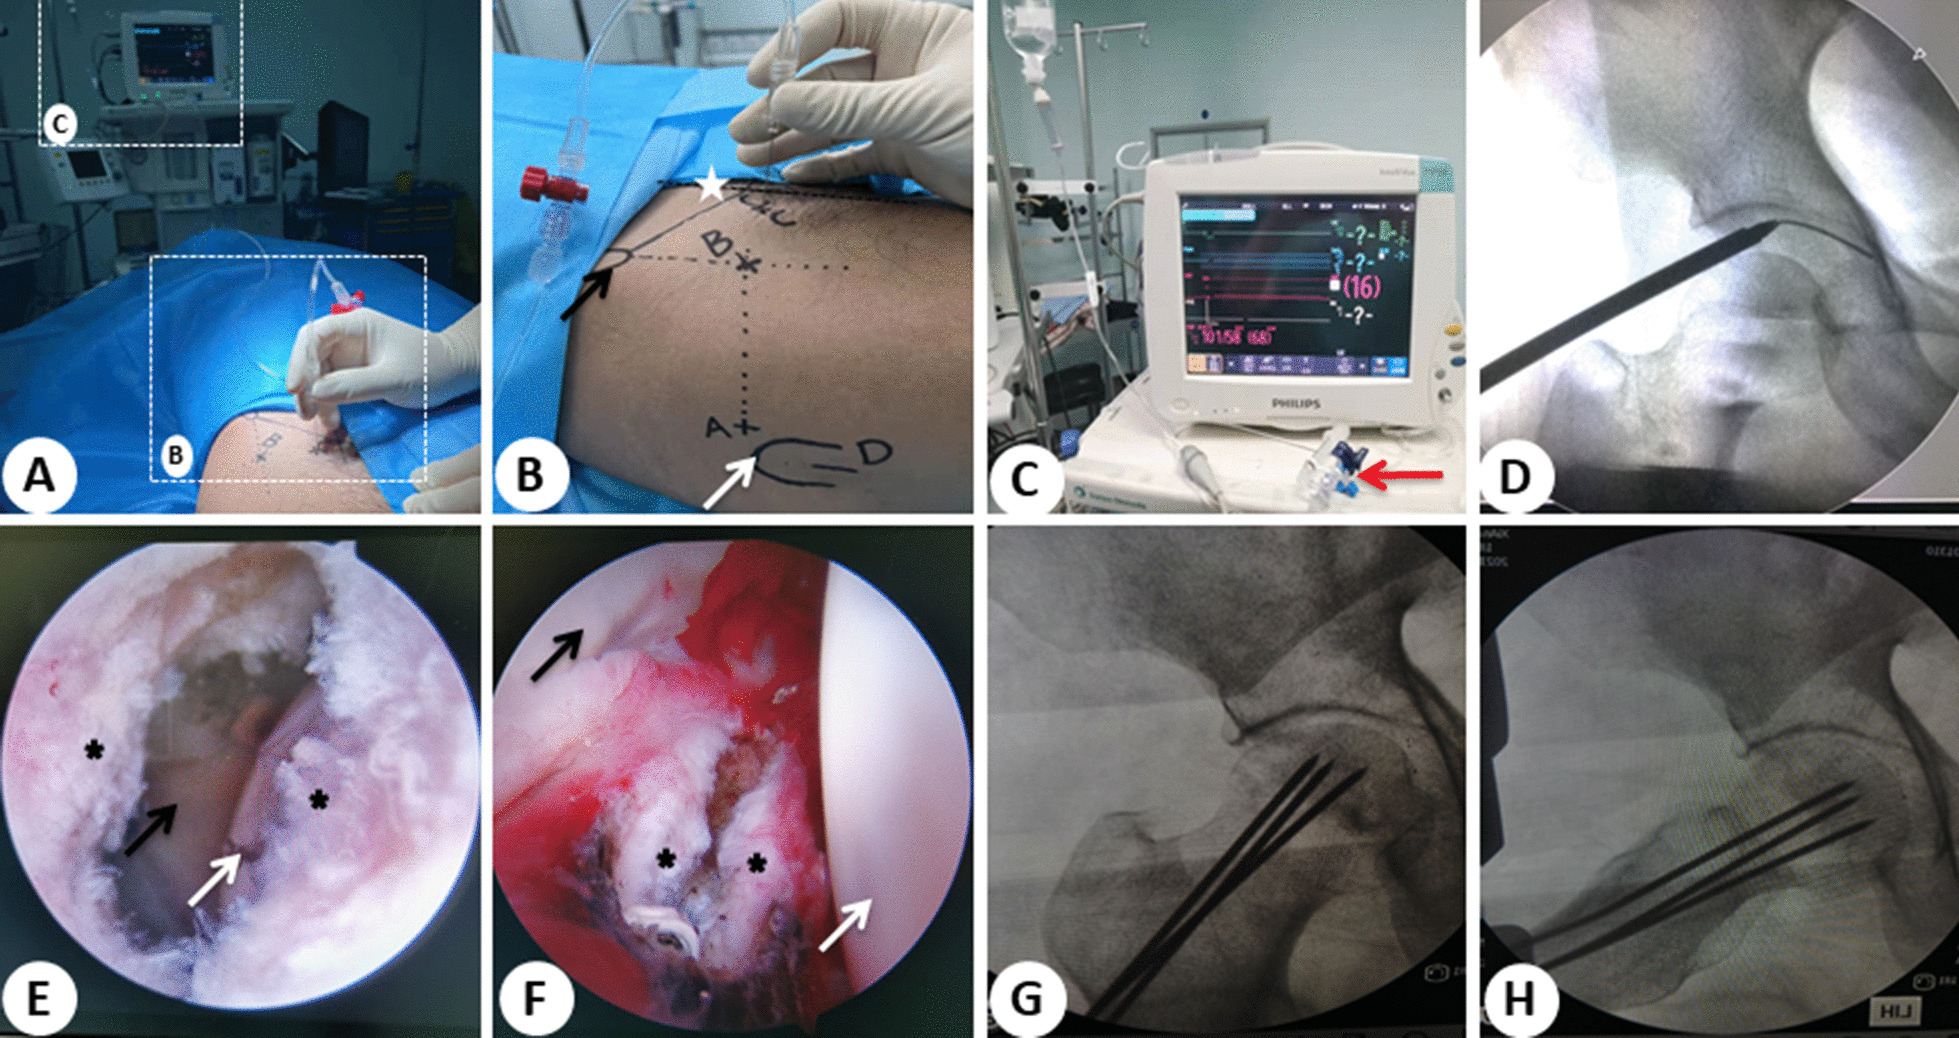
Measurement of intra-articular pressure and surgical operation. A Measurement of intra-articular pressure. B Bony landmarks and approaches of the hip joint (A/B/C/D symbol indicate the anterolateral approach, the anterior approach, the puncture point of intra-articular pressure measurement and the approach of core decompression, respectively. The black arrow indicates the anterior superior spine, the white arrow indicates the greater trochanter, and the white pentacle indicates the route of femoral vascular and nerve). C AI-4423 pressure sensor and IntelliVue MP50 pressure recorder. (The red arrow is a pressure sensor connected to the pressure detector.) D Establishment of the anterolateral approach for hip arthroscopy. E Incised lateral joint capsule outside the joint capsule through the view of the anterolateral approach. (The black pentacle shows the open joint capsule viewed from the outside, the black arrow represents the labrum, and the white arrow represents the femoral head.) F Incised lateral joint capsule inside the joint capsule through the view of the anterior approach. (The black pentacle shows the open joint capsule viewed from the inside, the black arrow represents the labrum, and the white arrow represents the femoral head.) G, H Intraoperative X-ray fluoroscopy of core decompression in the positive and frog positions
